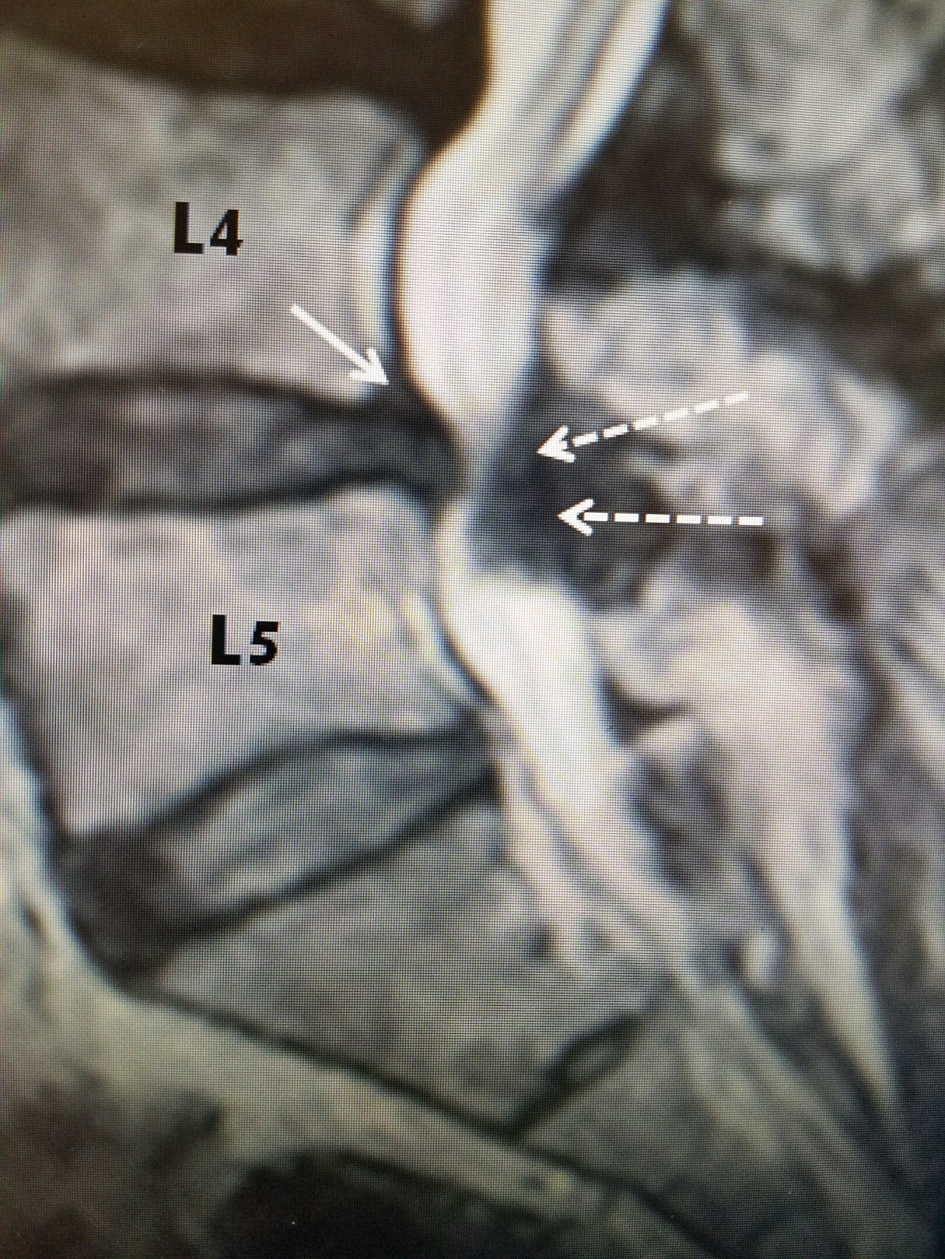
MRI of lumbar spine with L4-5 stenosis T2 sagittal MRI of L4-5 stenosis secondary to hypertrophied yellow ligament posteriorly (dashed white arrows) and minimal grade I L4-5 anterolisthesis with disc protrusion posteriorly (solid white arrow). Note that on T2 MRI, bone is a gray color and soft tissue, ligament, and disc annulus are darker. MRI: Magnetic resonance imaging.
